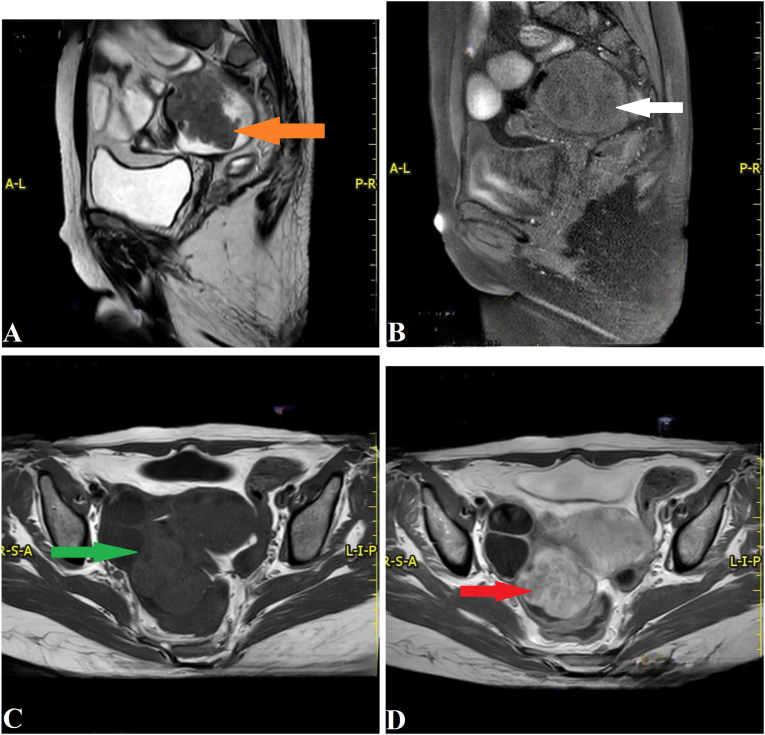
Pelvic magnetic resonance imaging (MRI). (A) Sagittal T2-weighted image showing a large polypoid mass with intermediate signal intensity (orange arrow). (B) Sagittal T1 fat-suppression pre-contrast image showing a mass with intermediate signal intensity (white arrow). (C) Axial T1-weighted image without contrast, demonstrating a mass with intermediate signal intensity (green arrow). (D) Axial T1-weighted post-contrast image showing avid enhancement of the tumor (red arrow). (For interpretation of the references to colour in this figure legend, the reader is referred to the web version of this article.)
